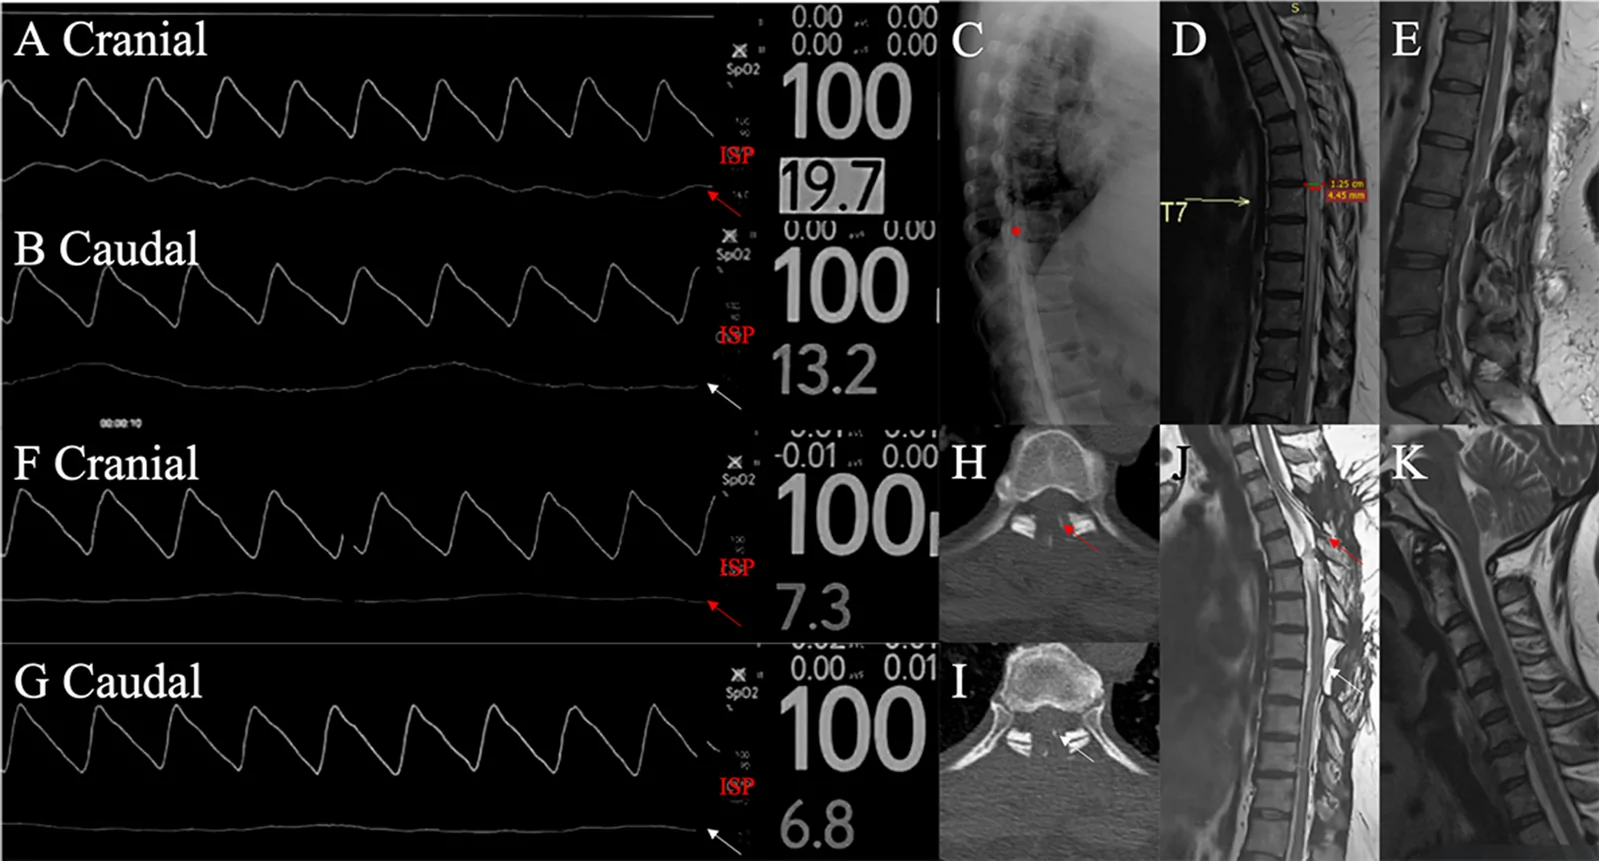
A 40-year-old female presented with progressive numbness and weakness in both lower extremities for over one year following a thoracic SCI, culminating in an inability to walk over the preceding three months. CASE1: (A, B) Preoperative intraspinal pressure (ISP) tracings cranial (A, red arrow) and caudal (B, white arrow) to the arachnoid scar show markedly elevated pressure gradient with superimposed abnormal waveform patterns. (C) Lumbar myelogram demonstrating a complete block at the T7 level due to dorsal arachnoid adhesion (red asterisk). (D, E) Preoperative T2-weighted magnetic resonance (MR) images reveal an intramedullary syrinx and spinal cord edema extending rostrally to T5. (F, G) Postoperative ISP tracings at corresponding locations (F: cranial, G: caudal) after S-S bypass placement demonstrate normalization of cranial waveforms and a significant reduction in pressure gradient. (H, I) Postoperative computed tomography (CT) images confirm the position of the S-S bypass conduit spanning from T4 (red arrow) to T7 (white arrow). (J, K) Postoperative T2-weighted MR images show marked regression of the syrinx and spinal cord edema. Supplementary video 1 shows the patient walking again after S-S bypass
